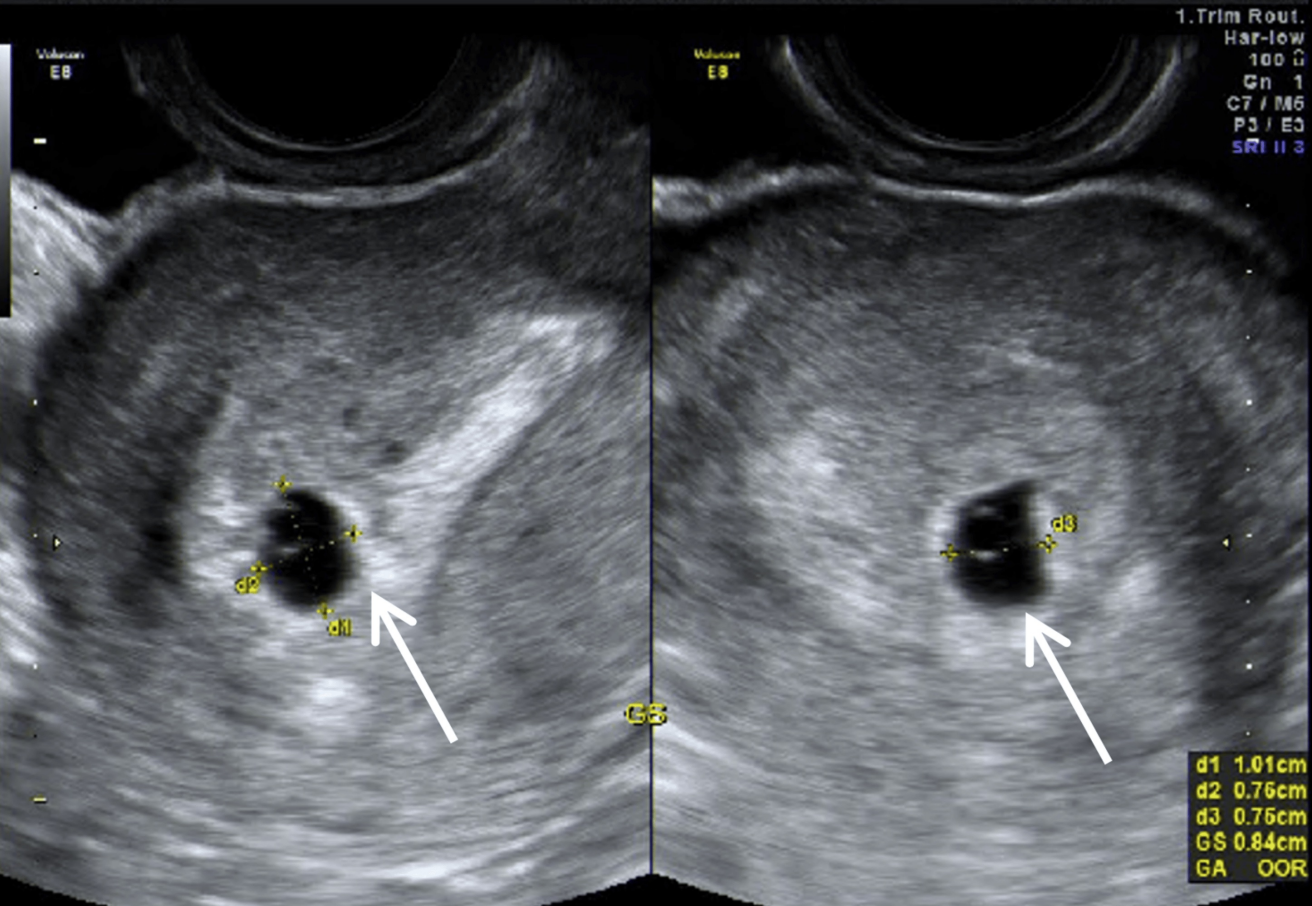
Transvaginal ultrasound 17 days after OPU The white arrow indicates an intrauterine gestational sac with a yolk sac. GS, gestational sac, measured in three dimensions (d1, d2, d3); OPU, oocyte pick-up
